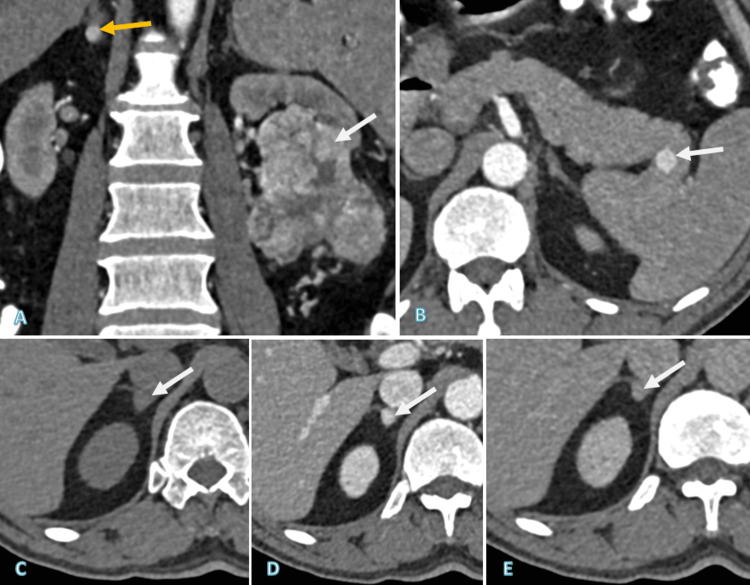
Left RCC metastasis – right adrenal with dissemination: coronal CECT (A) shows an irregular hyperenhancing left renal mass (white arrow) and a right adrenal nodule (yellow arrow) mimicking its enhancement. Axial CECT (B) reveals a synchronous, hypervascular metastatic nodule in the tail of the pancreas (white arrow). Axial CECT images show a right adrenal nodule (white arrow) with high unenhanced attenuation (C) and avid enhancement (D), demonstrating rapid washout (E) mimicking adenoma; however, enhancement parallels primary RCC, consistent with metastatic disease. RCC = renal cell carcinoma; CECT = contrast-enhanced CT
